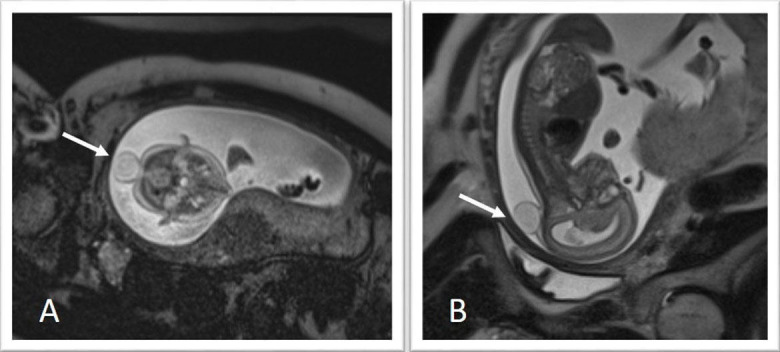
Meningocele Axial (A) and Sagittal (B) T2-weighted HASTE images demonstrate a cystic collection along the dorsal aspect of the occipital region, which is compatible with a meningocele.
